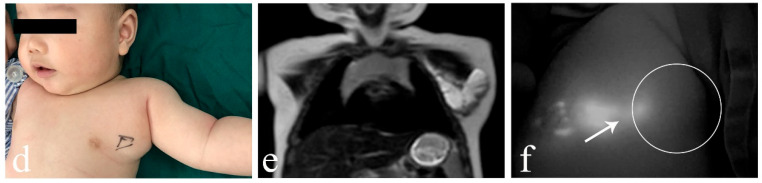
Images of macro/mixed cLM without ICH. (a) A 4-year-old female patient with macro cLM of the right neck. (b) Preoperative MRI indicated macro cysts without ICH. (c) Intraoperative ICG lymphography finding with one inflow from posterior auricular region draining into the lesion. (d) A 4-month-old female patient with mixed cLM of the left chest. (e) Preoperative MRI indicated mixed cysts without ICH. (f) Intraoperative ICG lymphography finding with one inflow from areola region draining into the lesion. *Arrow head*, lymph vessel; *circle*, region of the lesion. cLM, cystic lymphatic malformation; ICH, intracystic hemorrhage; MRI, magnetic resonance imaging; ICG, indocyanine green.
